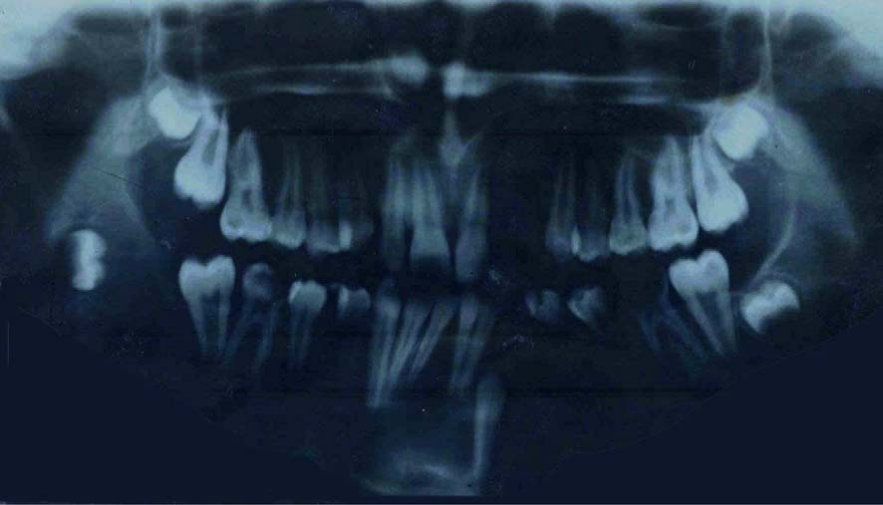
Dental Orthopantomography of a patient affected by NBCCS. (Patient n.11 of table 2).
